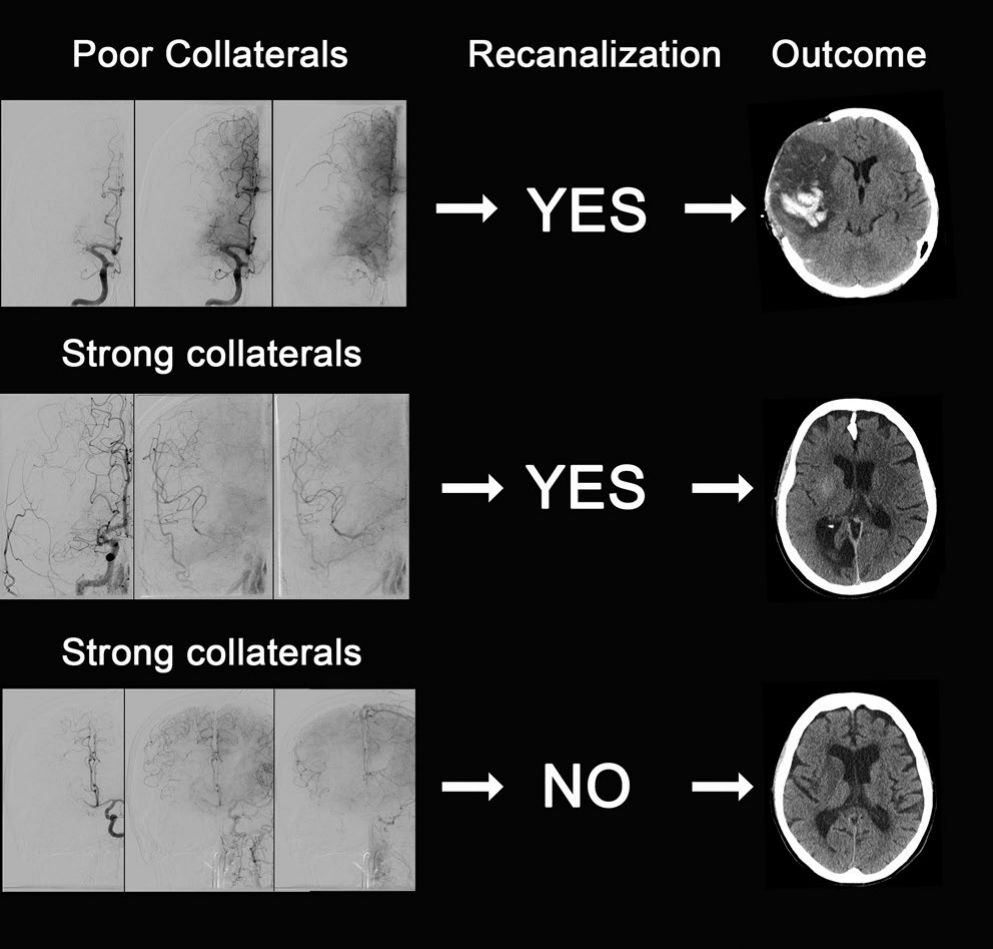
Strong association between collateral status at baseline and outcome. *Upper row from left to right*: Baseline frontal digital subtraction angiography (DSA) projection in arterial, parenchymal and early venous phase shows poor filling of the macrovascular pial arterial tree in right M1 occlusion. Despite successful recanalization (thrombolysis in cerebral infarction (TICI) 2b, not shown) unfavorable outcome with complete middle cerebral artery (MCA) infarction and hemorrhagic transformation ensued necessitating decompression hemicraniectomy (cranial computed tomography (CCT) on upper *right*). *Middle row*: Strong filling of pial collaterals at baseline in in right M1 occlusion. After successful recanalization (TICI 2b, not shown) favorable outcome was observed with only limited striatal infarction conspicuous by mild contrast extravasation. *Lower row*: Strong filling of pial collaterals at baseline in right internal carotid artery (ICA) occlusion (contralateral/*left* ICA injected). Even though recanalization could not be achieved in this case, neither by transfemoral access nor by direct carotid puncture, outcome was still favorable with only limited striatal infarction on follow-up CCT conspicuous by hypoattenuation (CCT on *lower right*)
